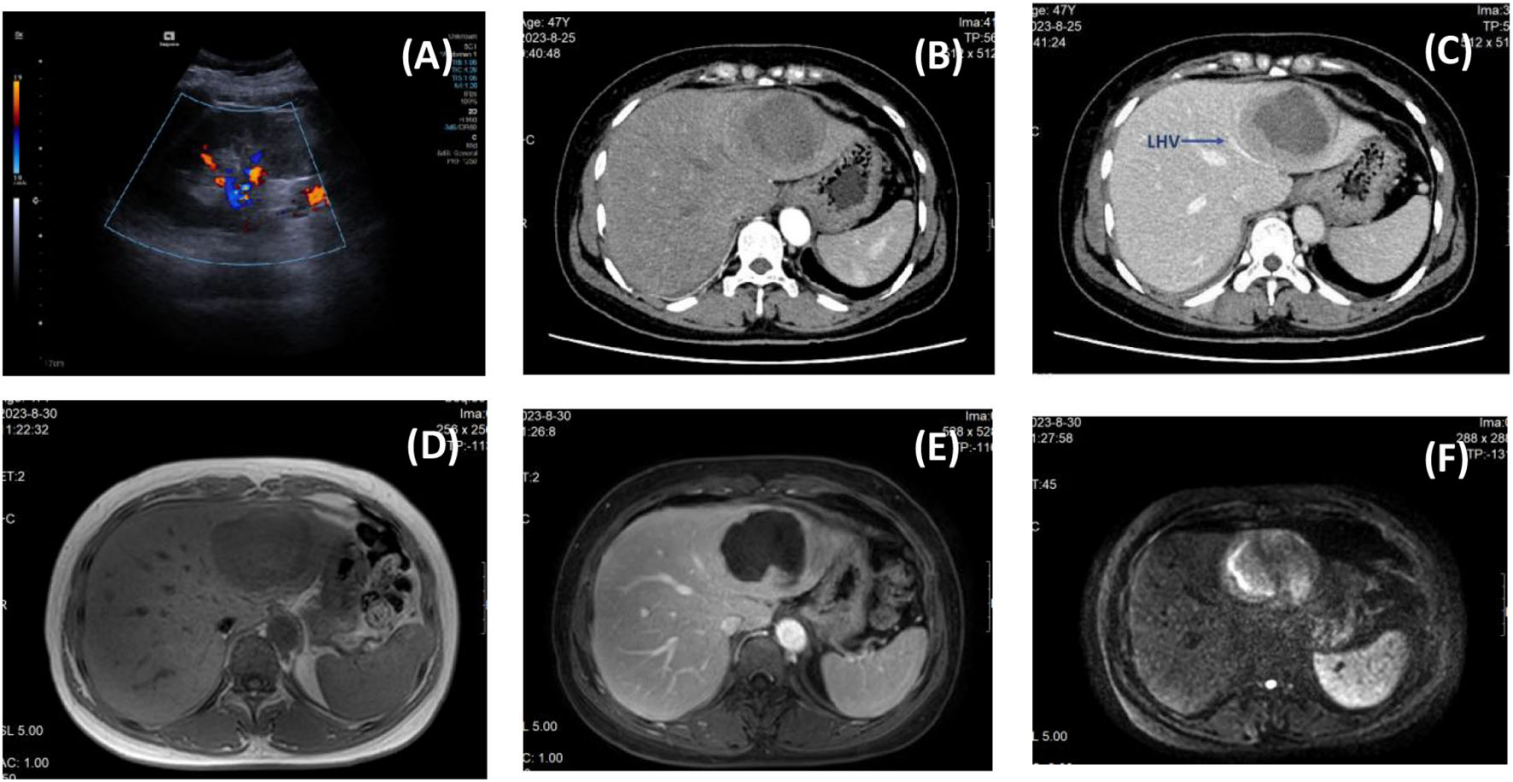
Preoperative imaging findings. [(A) B-ultrasound; (B) Arterial phase of enhanced CT; (C) Portal phase of enhanced CT, the blue arrow indicates the compressed left hepatic vein; (D) Fat imaging in MRI; (E) Arterial phase of MRI; (F) DWI image in MRI.].
